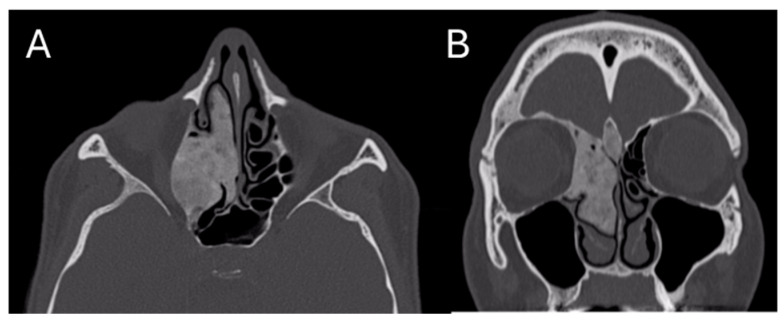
Pre-operative CT Image of Patient 1. (A) An axial cut; (B) a coronal cut. In both, the extension of the lesion into the ethmoidal region is evident.
